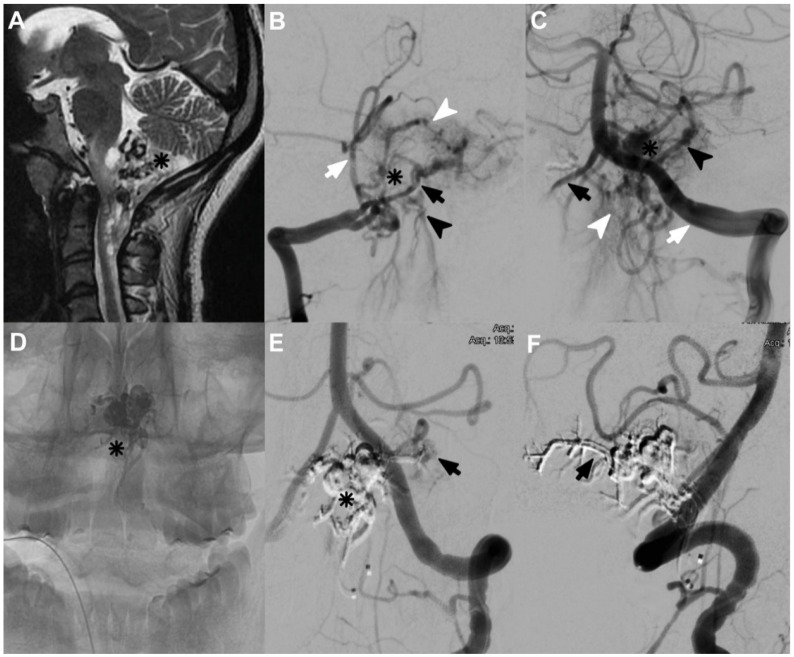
(A) The T2 MRI of a complex AVF of the CCJ (asterisk). (B) The AP DSA of the right vertebral artery (VA); an extracranial PICA is noted (white arrow), as is a small arterial aneurysm (asterisk). Hypoplasia of the V4 segment (black arrow) and an arterial shunt (white arrowhead) are also evident. Venous drainage towards the marginal sinus and the extradural venous plexus (black arrowhead) can also be observed. (C) The AP left VA (white arrow); the hypoplastic right VA is noted (black arrow). Furthermore, the intradural PICA (black arrowhead), the dysplastic aneurysm (asterisk), and the extradural venous drainage (white arrowhead) can be observed. (D) Histoacryl on fluoroscopy. (E) The obstructed CCJ AVF, including the aneurysm (asterisk), is shown, but a small residual component can be noted (black arrow). (F) The left VA, showing the patency of the PICA, but a complete occlusion of the posterior meningeal artery (black arrow).
